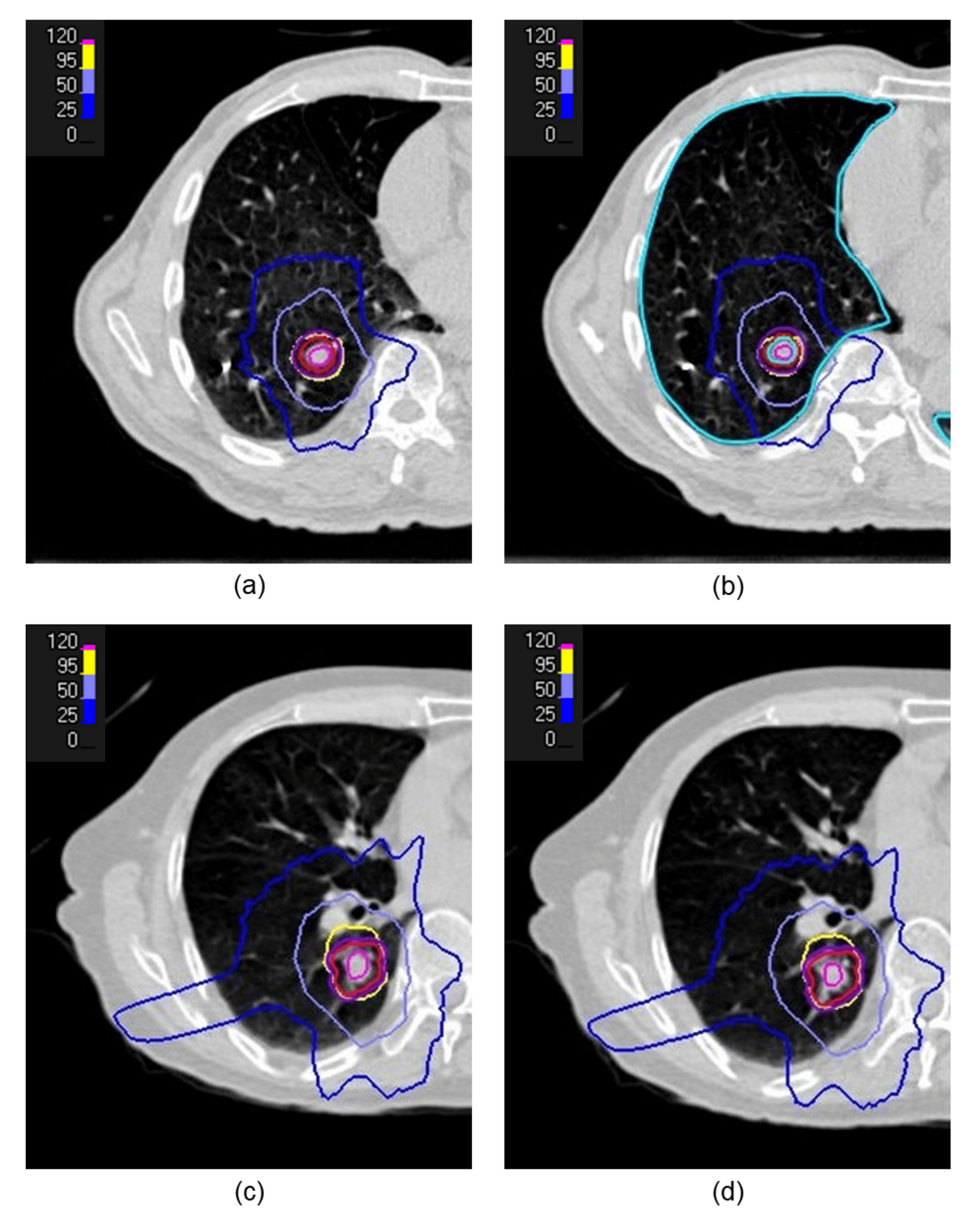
Dose distributions for Patient 1 and Patient 2 Dose distributions for Patient 1: (a) reference plan and (b) 4DCT-based plan at the 50% inspiratory respiratory phase. Dose distributions for Patient 2: (c) reference plan and (d) 4DCT-based plan at the maximum inhalation phase. The CTV is shown in red, and the PTV in dark purple. The 25%, 50%, 95%, and 120% isodose levels, relative to the prescribed dose, are shown in blue, light blue, yellow, and purple, respectively. CTV, clinical target volume; PTV, planning target volume; 4DCT, four-dimensional computed tomography
